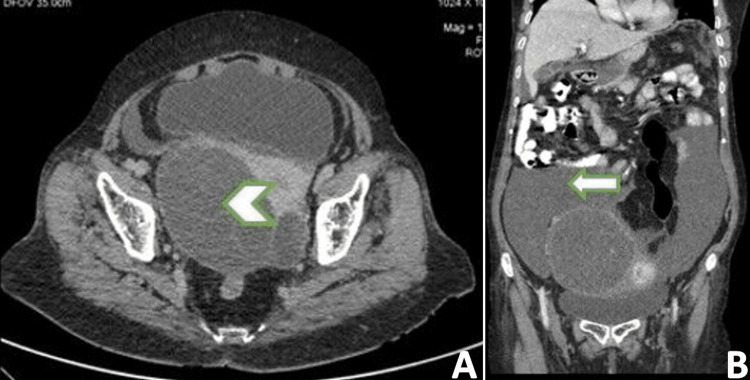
Contrast-enhanced computed tomography of abdomen and pelvis: (A) axial view and (B) coronal view CECT of abdomen and pelvis showed (A) a large peripherally enhancing cystic mass arising from the right ovary with thin enhancing septae (arrowhead); (B) mucinous fluid within the peritoneal cavity (white arrow).
